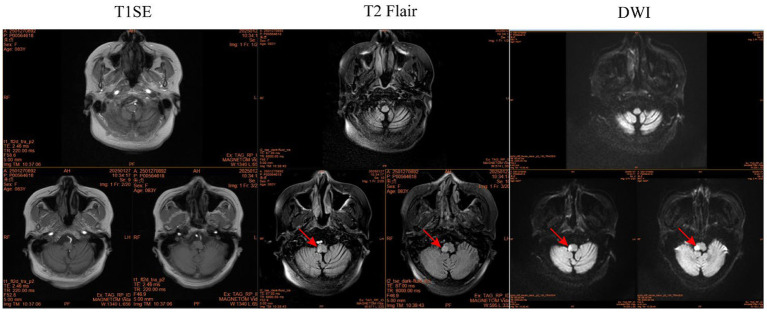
Head MRI on February 9 showed a right medulla oblongata subacute infarction. Axial diffusion-weighted imaging (DWI) sequence shows high signal intensity in the right medulla oblongata (arrow), indicative of acute infarction. Axial fluid-attenuated inversion recovery (FLAIR) sequence reveals multiple lacunar lesions in the bilateral basal ganglia (arrowheads).
